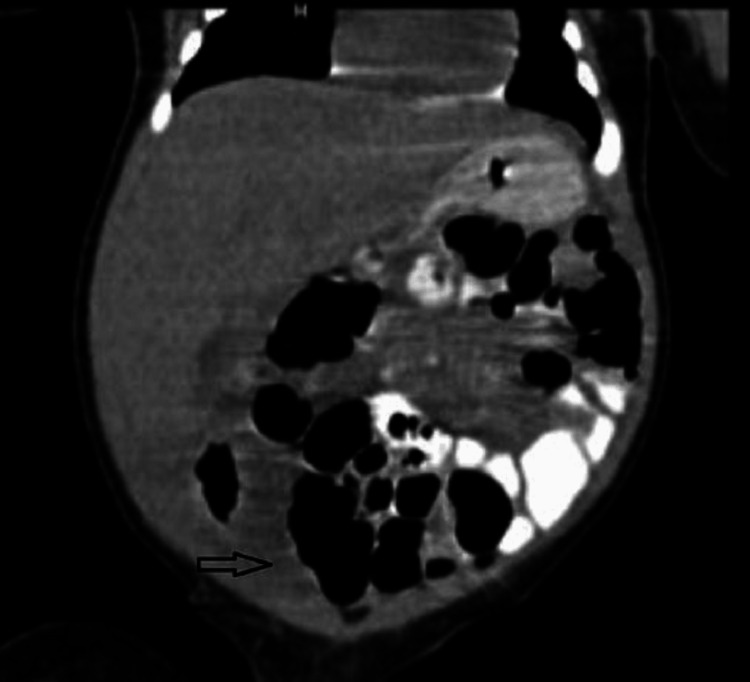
Contrast CT of the abdomen showing fluid in the right iliac fossa region (arrow). CT, computed tomography
